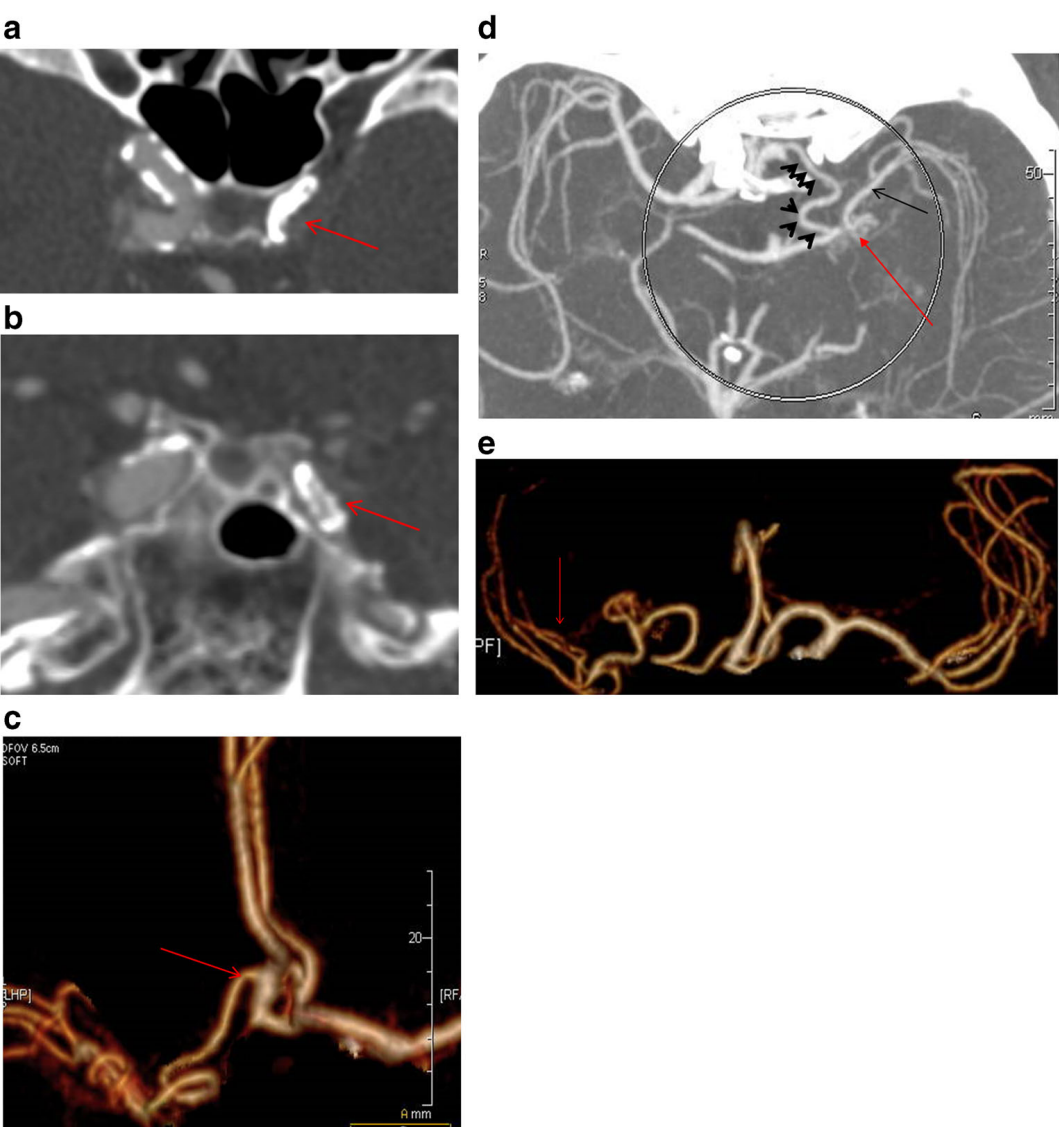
a Axial CT image at the level of the cavernous carotids shows occlusion of the left ICA (*red arrow*) due to atherosclerotic disease. b Coronal reconstruction at the level of the cavernous carotids verifies the occlusion of the left ICA (*red arrow*) as well as atherosclerotic disease. c CTA (VRT 3D reconstructions) shows the accessory MCA as a vessel coming from the A2 segment of the ipsilateral anterior cerebral artery (*red arrow*). d Axial MIP image shows the course of the AccMCA (*black arrowheads*), the anastomotic network (moyamoya type) at the level of the left mid M1 segment (*red arrow*) as well as the patent peripheral left MCA (*black arrow*). e CTA (VRT 3D reconstructions) reveals the same configuration and patent peripheral MCA (*red arrow*)
